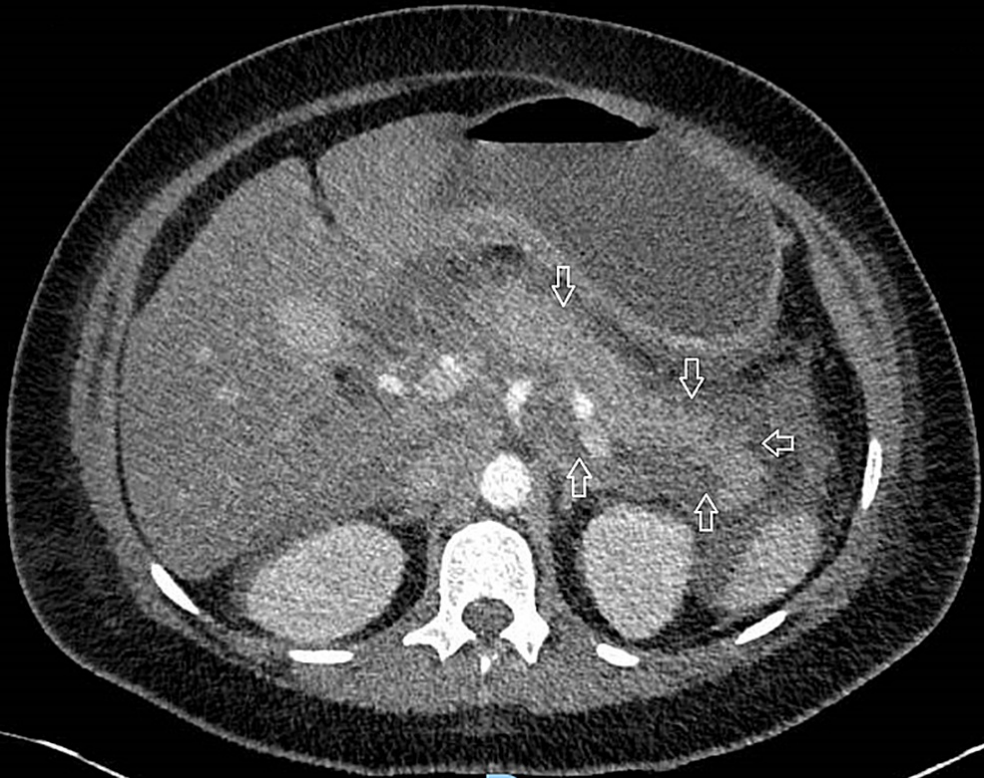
CT scan of the abdomen and pelvis Peripancreatic fat is infiltrated (arrows) and there is a fluid surrounding the pancreas and along with the lesser sac consistent with acute pancreatitis.
